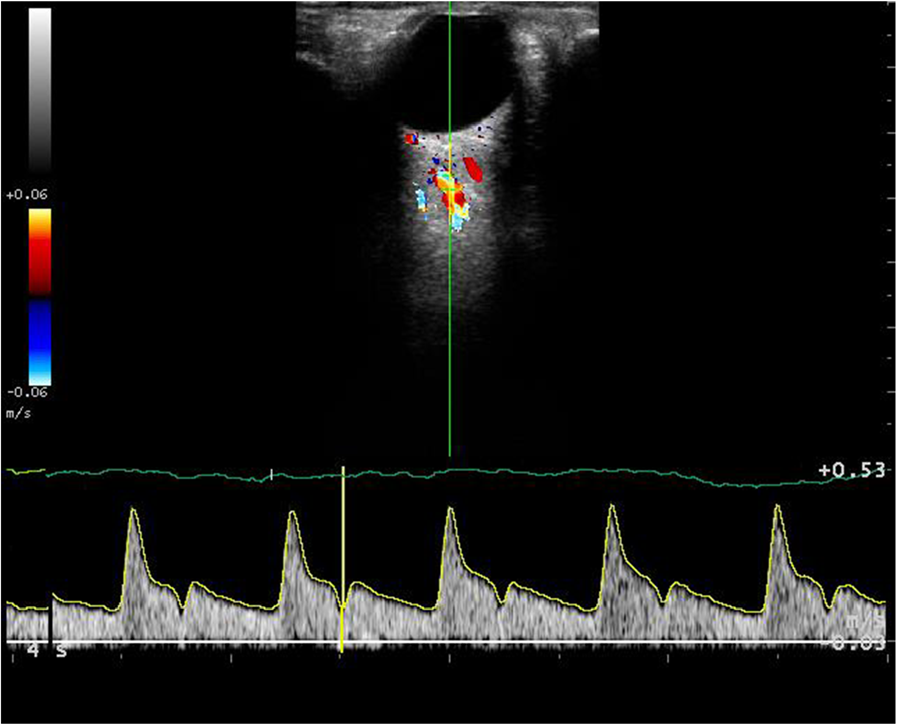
Doppler ultrasound of right ophthalmic artery.
